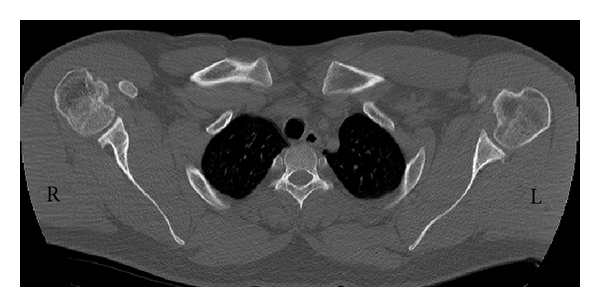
One-year follow-up axial CT scan view shows bilateral small defect (less than 25%) in anteromedial part of the humeral head. These defects are less than what was seen in Figure 2.
